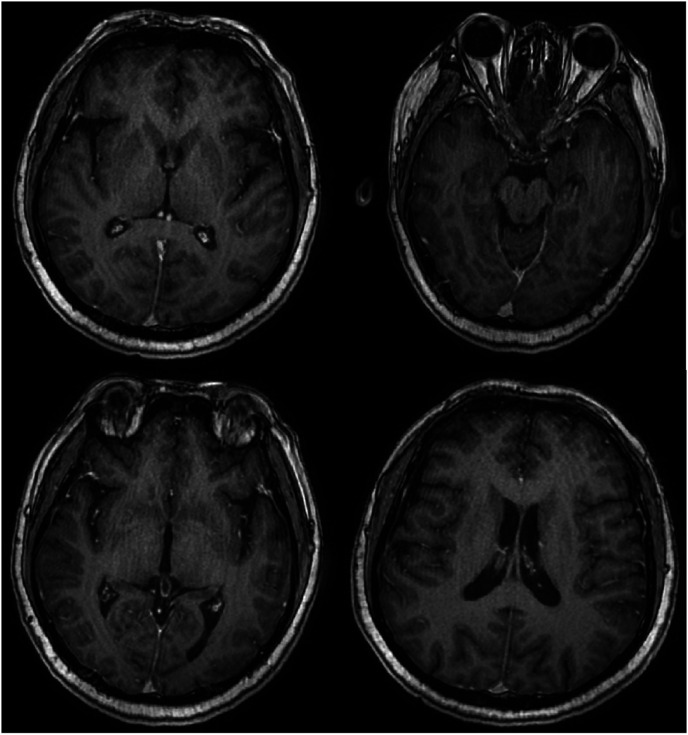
Magnetic resonance imaging of the brain using the CUBE sequence, showing no abnormal enhancement foci.
